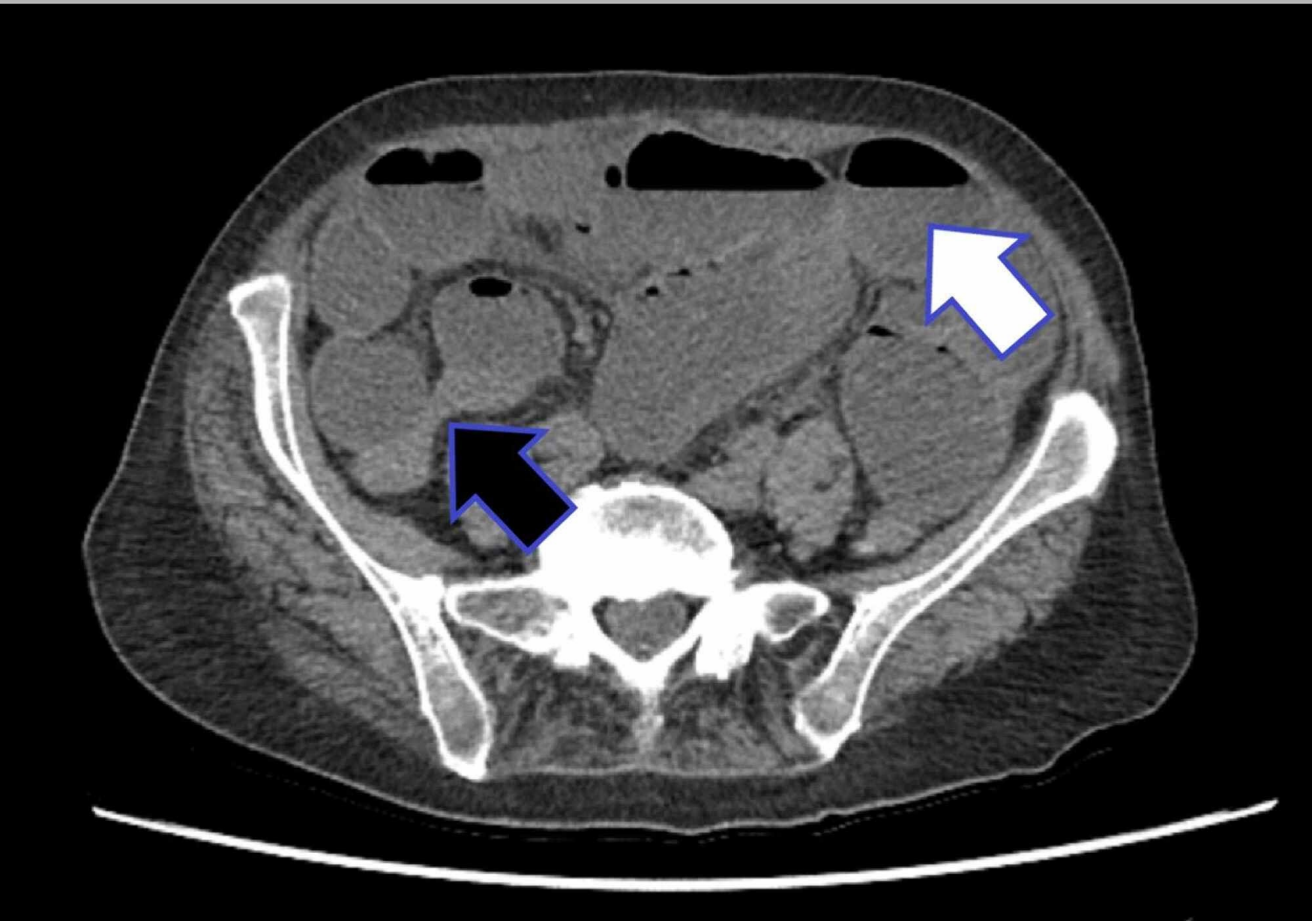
An axial cut CT scan showing small bowel dilation consistent with small bowel obstruction (white arrow indicates distended, fluid- and gas-filled small bowel; black arrow indicates the transition point at the ileocaecal valve)
